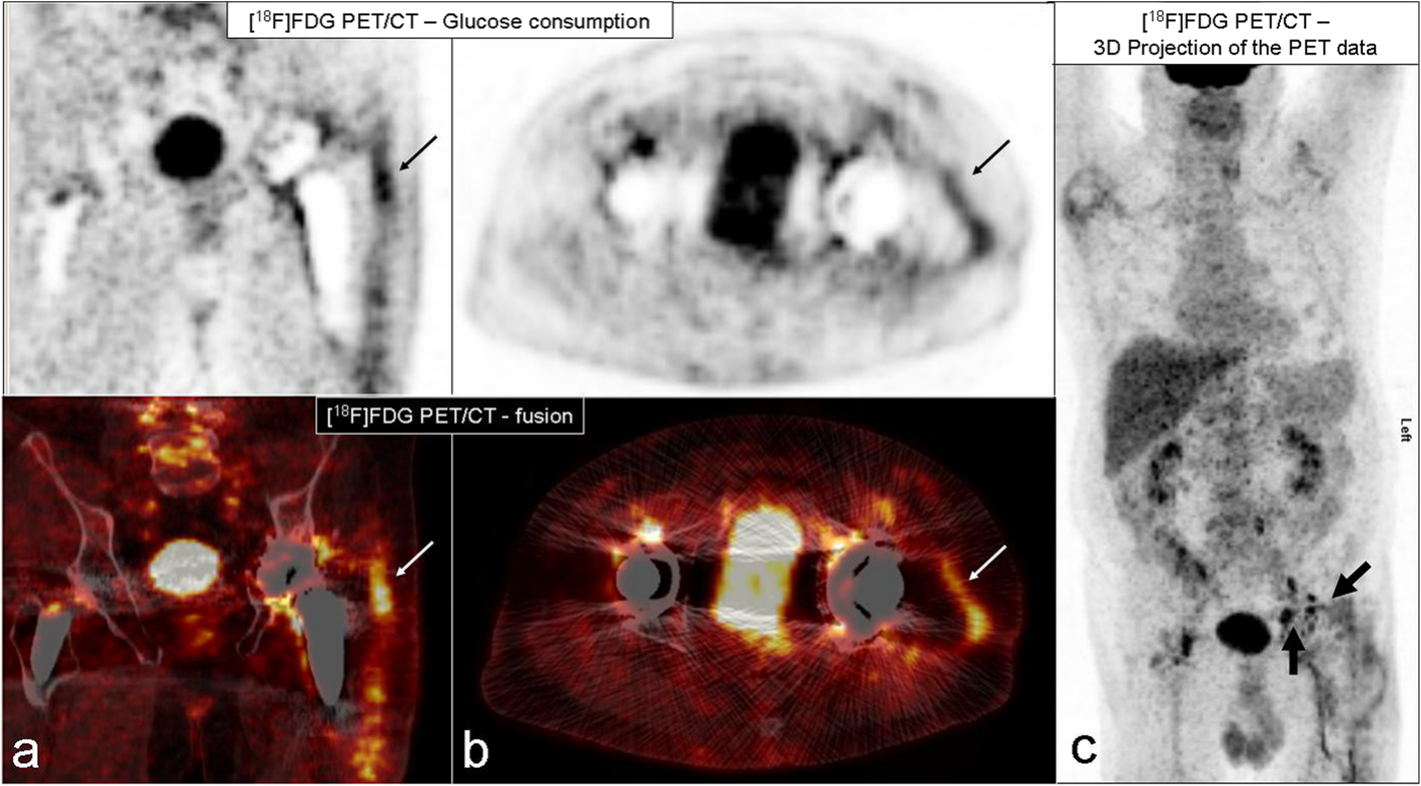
PET/CT images. Representative [18 F] FDG PET/CT images of a patient with infection of the left total hip arthroplasty in coronal (a) and transversal (b) orientation (c) shows a 3D projection (maximum intensity projection) of the [18 F] FDG PET data. The images demonstrate a streaky intensive hypermetabolism in the soft parts surrounding the total hip arthroplasty (thin arrows) and focal hypermetabolisms in reactive inguinal lymph nodes (bold arrows)
